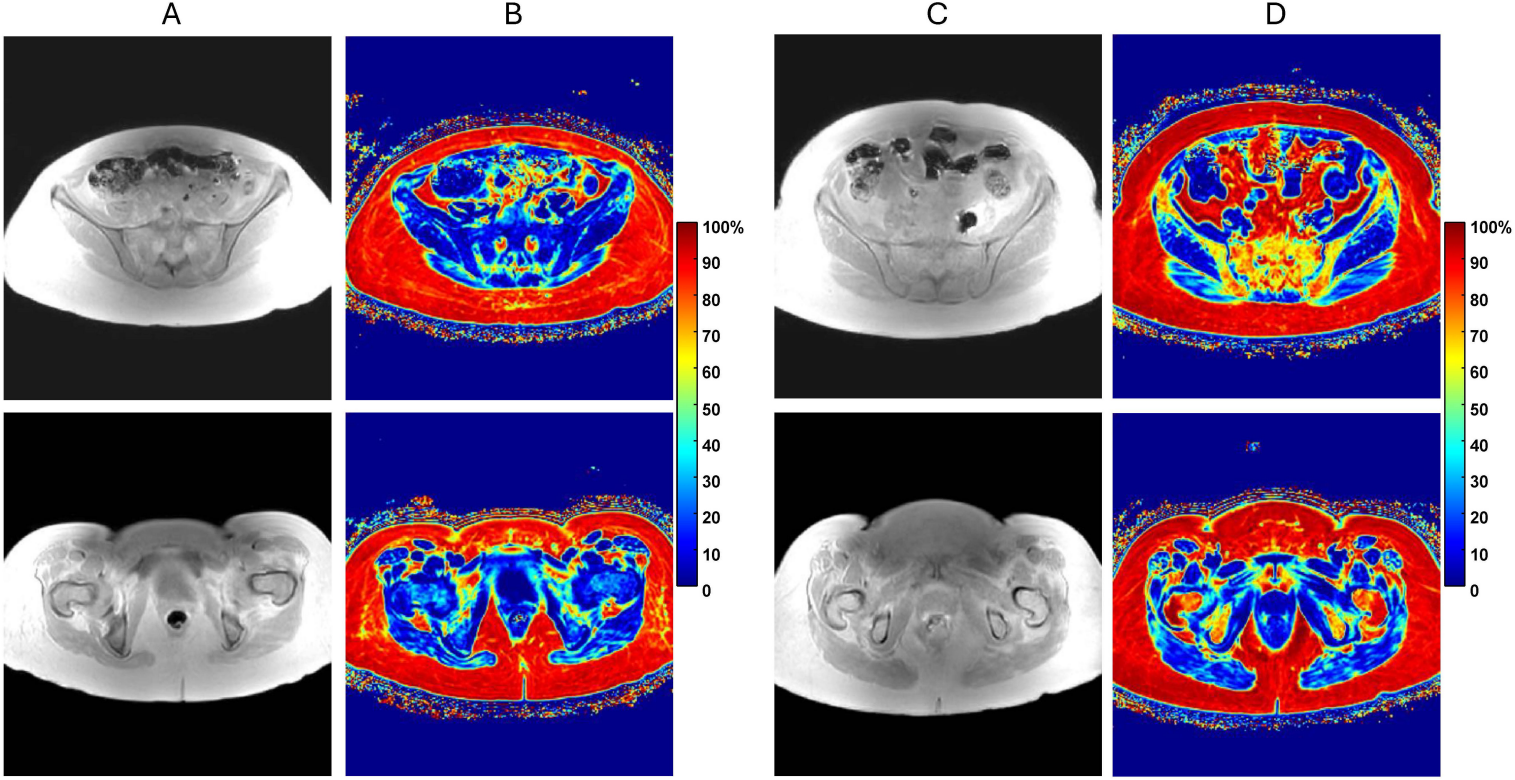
(A) In-phase (IP) pelvic MRI of myelofibrosis (MF) patient with low bone marrow fat content for axial slice through ilium (top) and femur (bottom). (B) Corresponding quantitative proton-density fat-fraction (PDFF) maps shown on 0 to 100% color scale. (C) Similar IP MRI of MF patient with relatively high fat content with (D) associated PDFF maps. Note, only IP images were used for segmentation by expert annotator and DL models.
